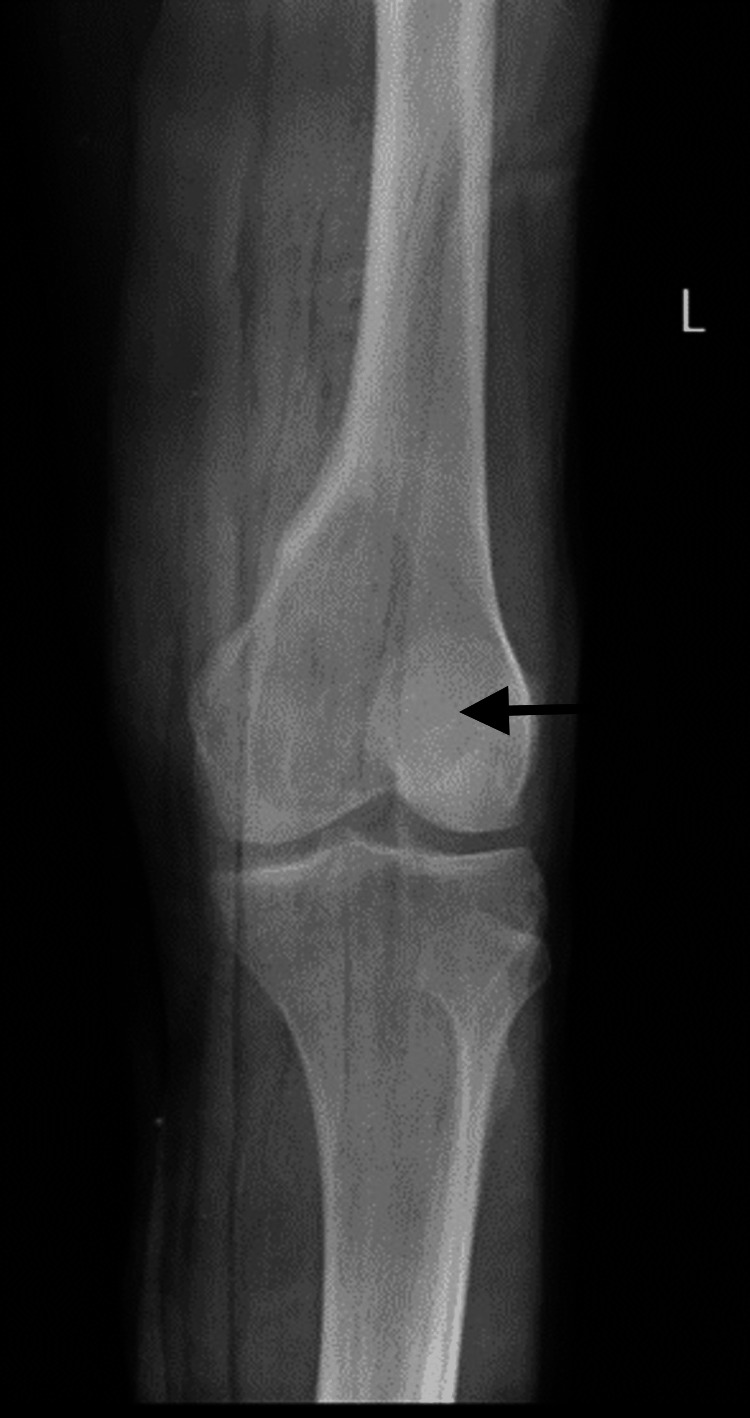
Plain radiograph of the knee, anteroposterior view shows lytic lesion over the lateral femoral condyle
